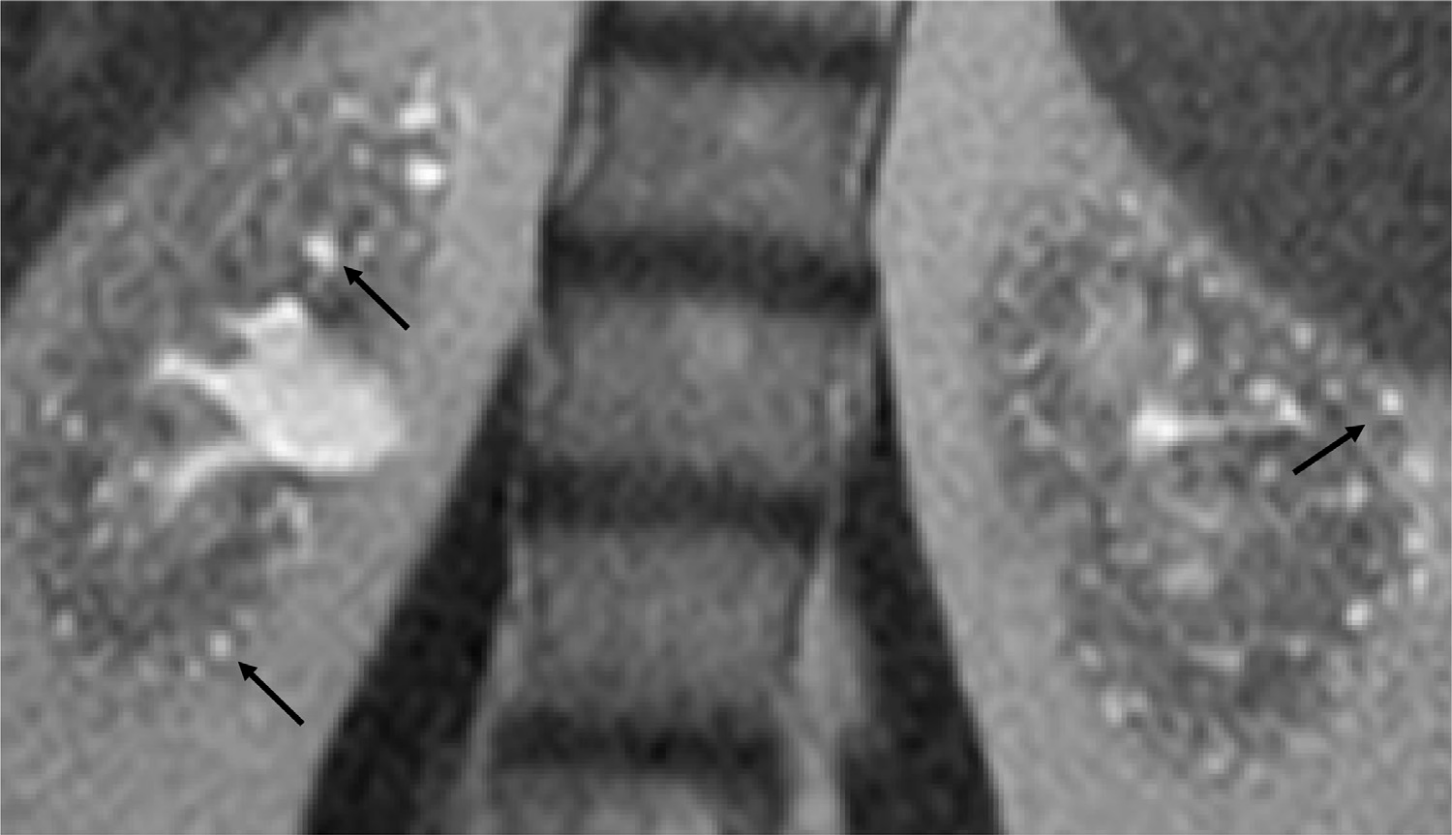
Select coronal T2 slice from MRI demonstrates normal-sized kidneys with multiple 1-2 mm sized T2 hyperintense renal cysts (black arrows) located within both the renal medulla and cortex.
